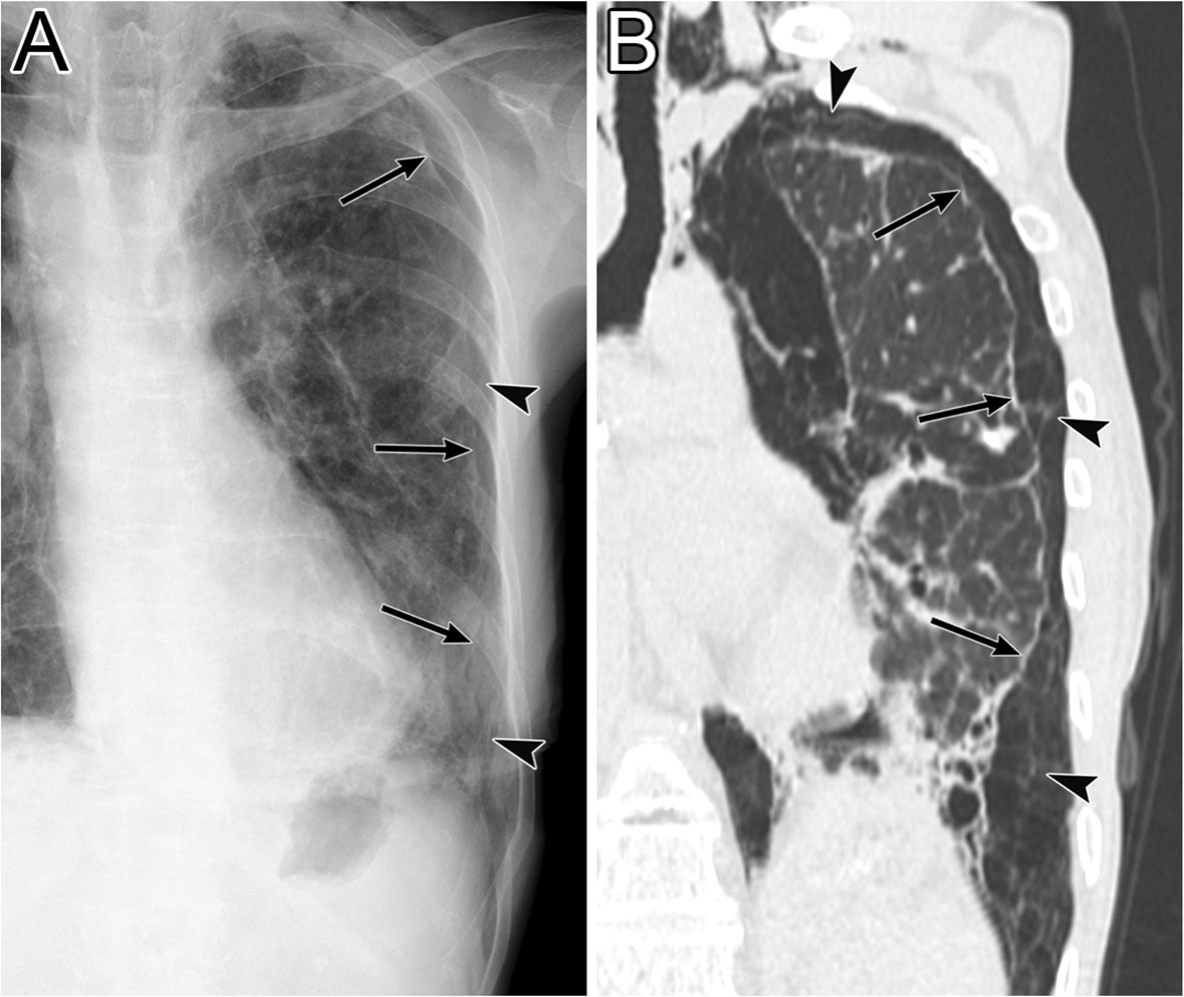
Air collection in the intrathoracic extrapleural space of a 64-year-old woman. She has Sjögren’s syndrome and interstitial pneumonia. a A supine radiograph shows a linear opacity (black arrows) in the left peripheral lung field that resembles pneumothorax. Fascial webs (black arrowheads) are visible outside of the linear opacity; this finding proves that air does not exist in the pleural cavity but collects in the intrathoracic extrapleural space. The linear opacity is composed of both the visceral and parietal pleura. Pneumomediastinum and subcutaneous emphysema are also visible. b A coronal CT image clearly depicts fascial webs (black arrowheads) in the intrathoracic extrapleural space. The mixture of the visceral and parietal pleura (black arrows) is slightly wavier than the typical visceral line in pneumothorax
